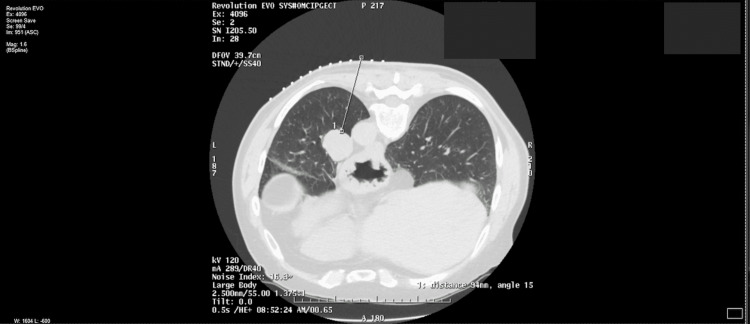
Computed tomography scan showing pulmonary carcinoid tumor prior to surgical resection
